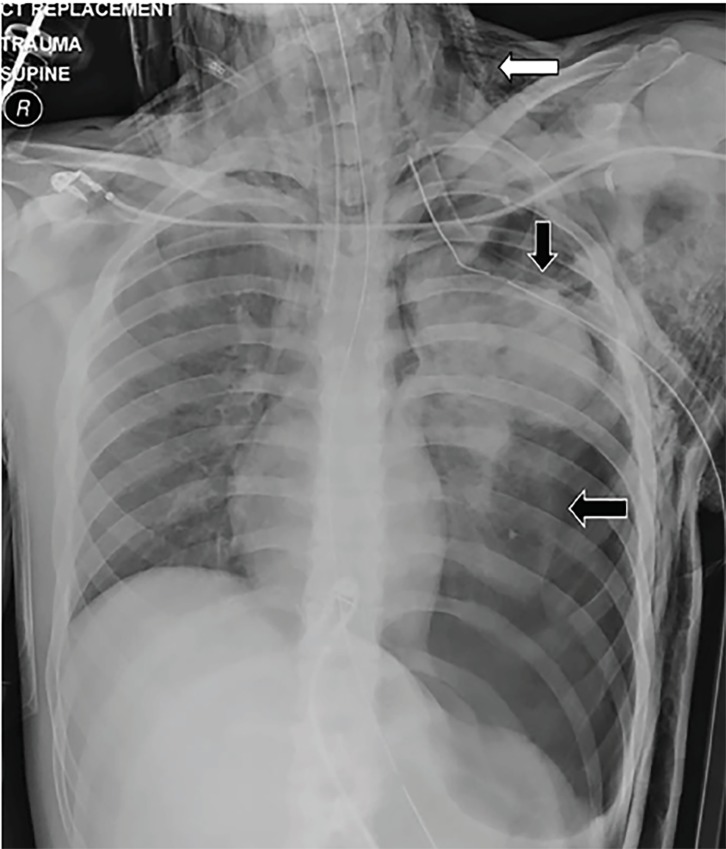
Chest radiograph demonstrating left tension pneumothorax (horizontal black arrow) with near- complete collapse of the left lung as well as significant subcutaneous emphysema (horizontal white arrow) to the left chest wall and neck. Also noted is a chest tube to the left chest (vertical black arrow).
